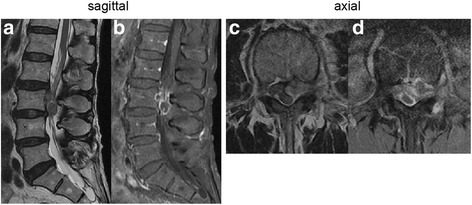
Preoperative MRI of the lumbar spine without and with intravenous contrast. T2-weighted MRI in the sagittal and axial planes showed a posterior epidural mass extending from the left past the midline to the contralateral posterior side (a and c). Fat-suppressed T1-weighted MRI in the sagittal and axial planes showed a lesion with a heterogeneous ring-like enhancement at the L3 level and demonstrated a sequestered fragment with posterior and superior migration in the dural sac (b and d)
